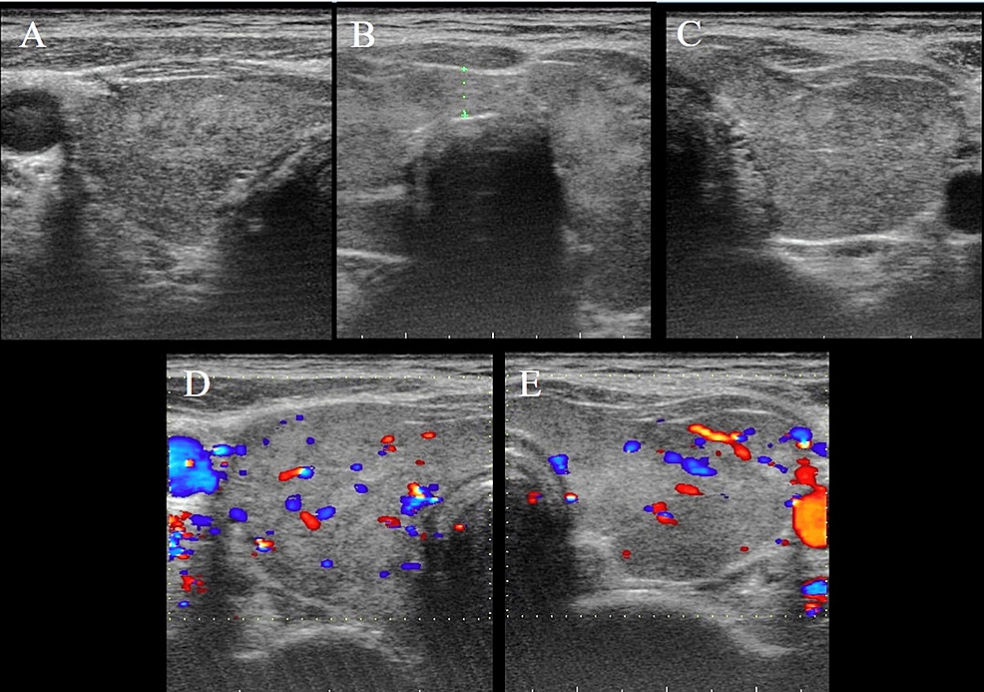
The result of thyroid ultrasonography. Thyroid ultrasonography (US) showing diffuse thyroid enlargement including the isthmus (A-C, A: right lobe, B: isthmus, C: left lobe). Color flow Doppler enhancement of the US images showing no increase in blood flow (D-E, D: right lobe, E: left lobe).
